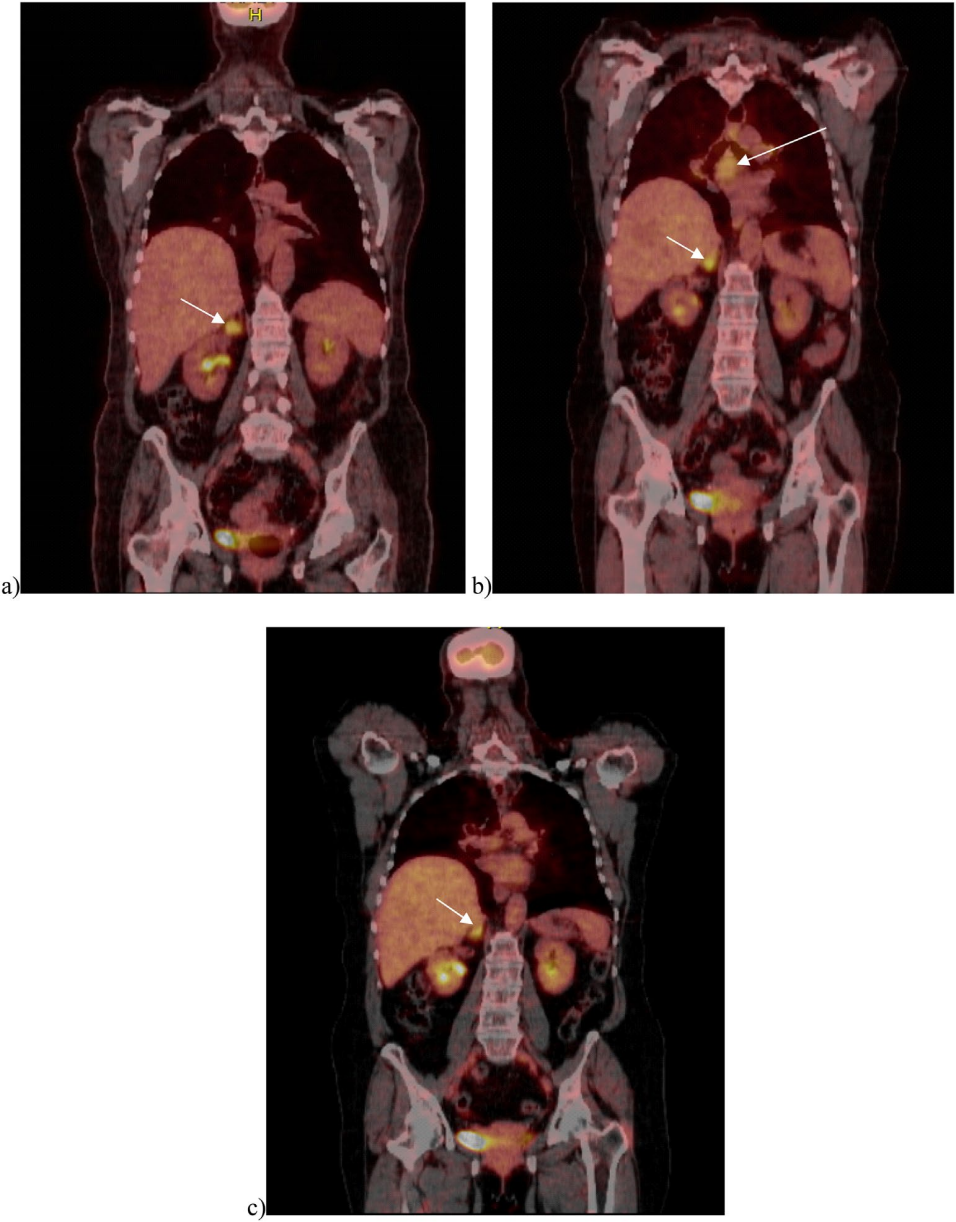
FDG‐PET/CT. (a) Initial FDG‐PET/CT revealed increased activity in the right adrenal mass with no additional site of uptake in coronal representation. (b) Follow‐up FDG‐PET/CT performed 3 months after the completion of immunotherapy showed a mild interval increase in the nodularity of the remaining part of the right adrenal gland with moderate uptake and interval increase in mediastinal nodal disease in coronal representation. (c) Follow‐up FDG‐PET/CT performed 3 months later showed complete resolution of mediastinal lymph nodes and a right adrenal showing normal physiological activity in coronal representation. No magnification of any of the images was done.
